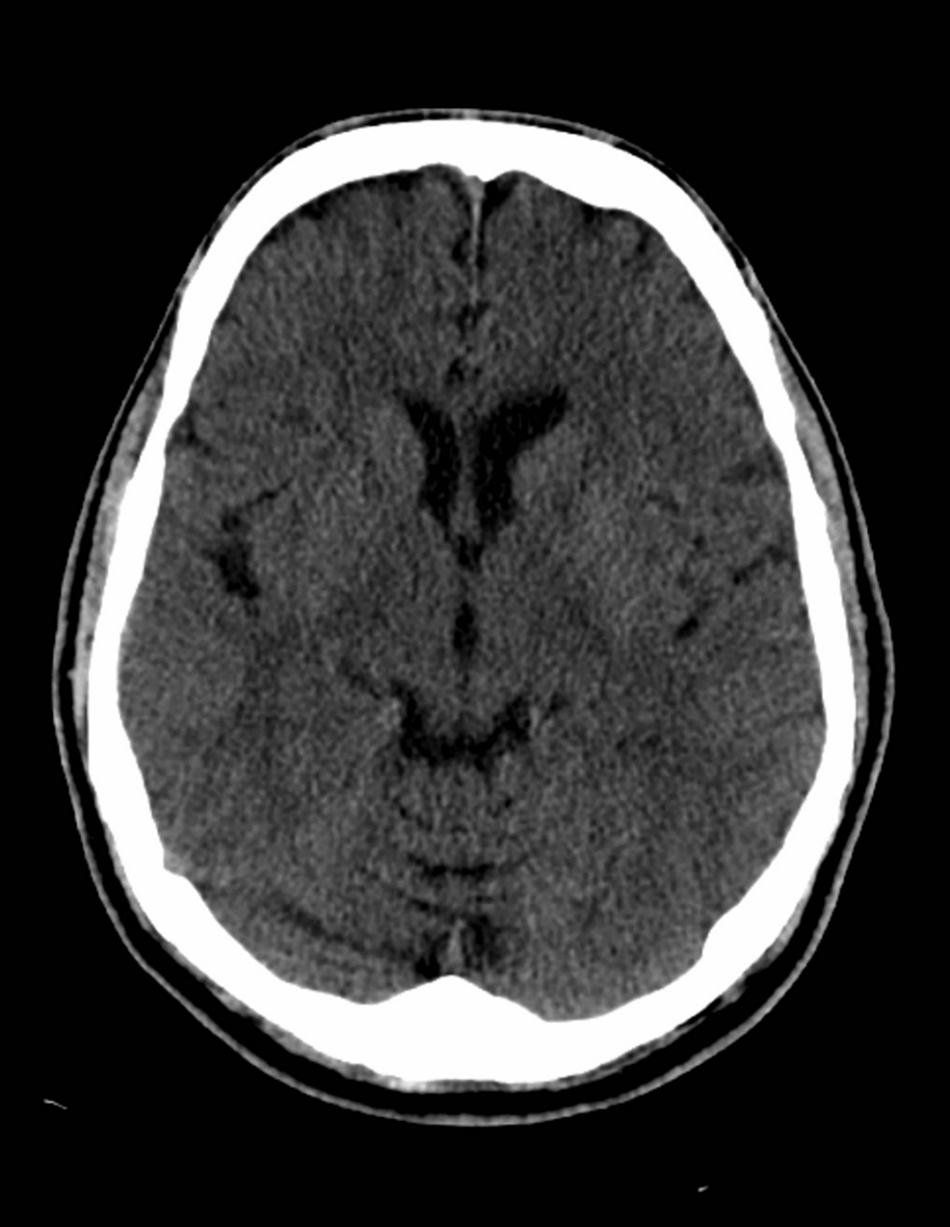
CT of the head without contrast showing no acute intracranial pathology.
